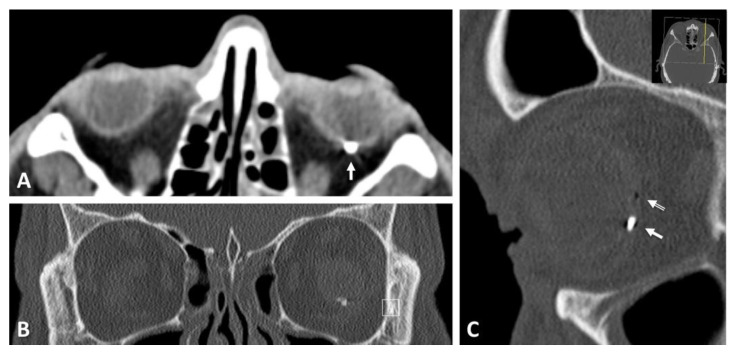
Computed tomographic images from the same patient mentioned in Figure 4. Frontal (A), axial (B) and sagittal (C) images, location of the metallic foreign body at the posterior pole (white arrow).
